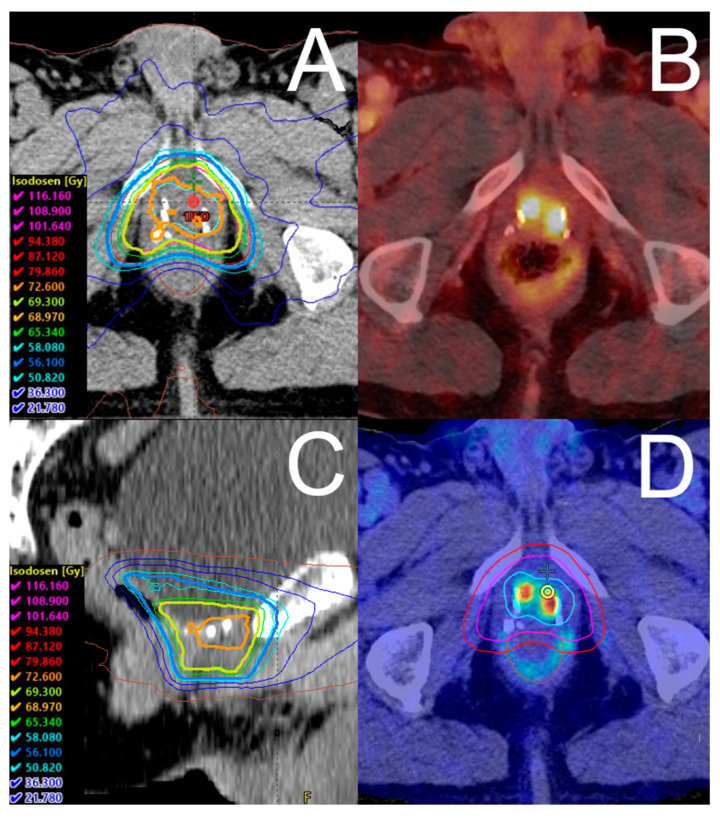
Exemplary dose distribution of salvage radiotherapy. Shown is an exemplary dose distribution in a patient receiving ^18^F-PSMA-1007 PET/CT-guided salvage radiotherapy in (A) axial view and (C) sagittal view. The corresponding ^18^F-PSMA-1007 PET/CT shows a PET-positive recurrence in the prostatic bed (B). The patient received volumetric modulated arc therapy with a simultaneous integrated boost to the local recurrence in three dose levels. (D) shows the fused PET/CT and corresponding radiotherapy contours. In 33 fractions, we prescribed single doses of 1.7 Gy for the PTV (red line), 2.1 Gy for the PTV_Boost1_ (pink line), and 2.2 Gy for the PTV_Boost2_ (turquoise line), resulting in total doses of 56.1 Gy (blue isodose), 69.3 Gy (bright green isodose), and 72.6 Gy (orange isodose), respectively.
